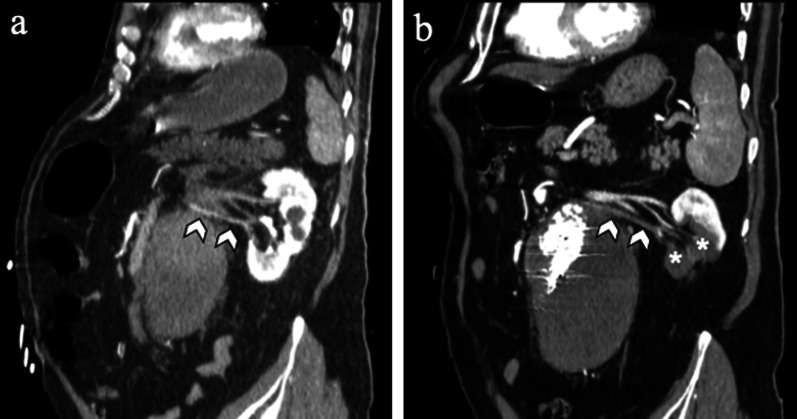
68-year-old man with segmental renal ischemia. a Sagittal CT angiography image shows left kidney normally perfused by the main left renal artery (not showed) and by an accessory renal artery (arrowheads); b sagittal CT image obtained from the same patient after EVAR shows occlusion of the accessory renal artery and lack of enhancement in the lower renal pole (*), which appears reduced in volume, due to chronic ischemia
